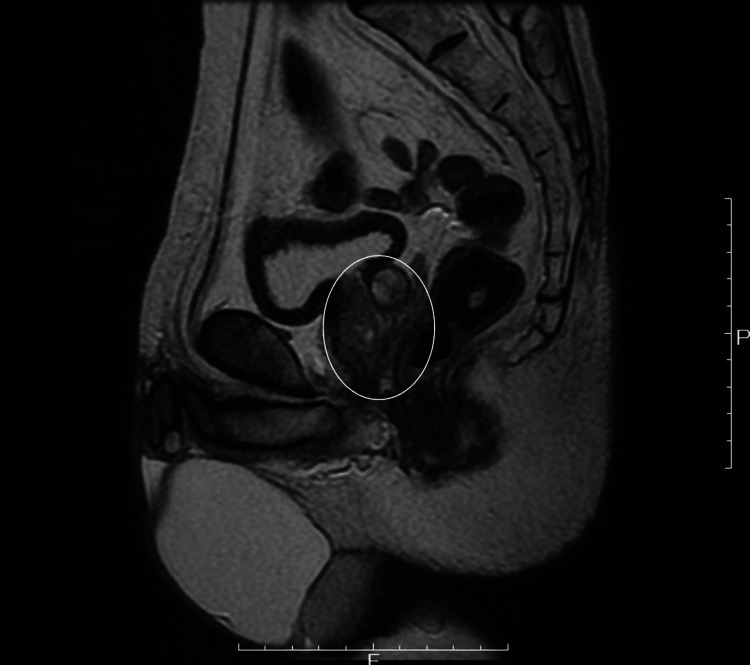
Sagittal MRI findings The white circle represents the prostate. No prostate enlargement was observed.
